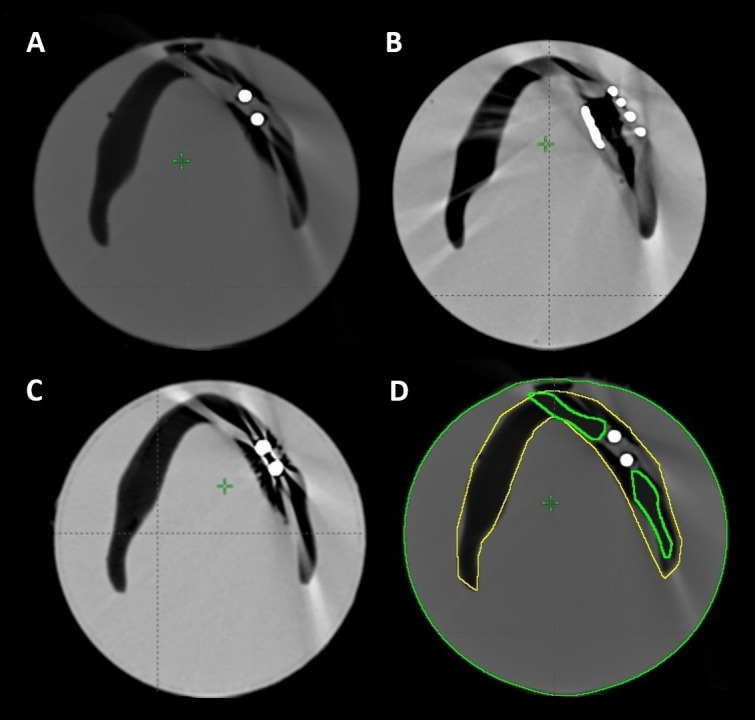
Representative axial CT slices demonstrating relative artifact severity and density management across different implant configurations: (A) Titanium endosseous implant, (B) Customized subperiosteal implant framework, (C) Zirconia implant exhibiting the most severe streak artifacts with a peak value of 38,059 HU, and (D) Visual demonstration of the artifact management protocol, where artifact-induced artificial air cavities in the adjacent tissue were manually contoured and assigned normal tissue HU values to ensure dosimetric accuracy.
